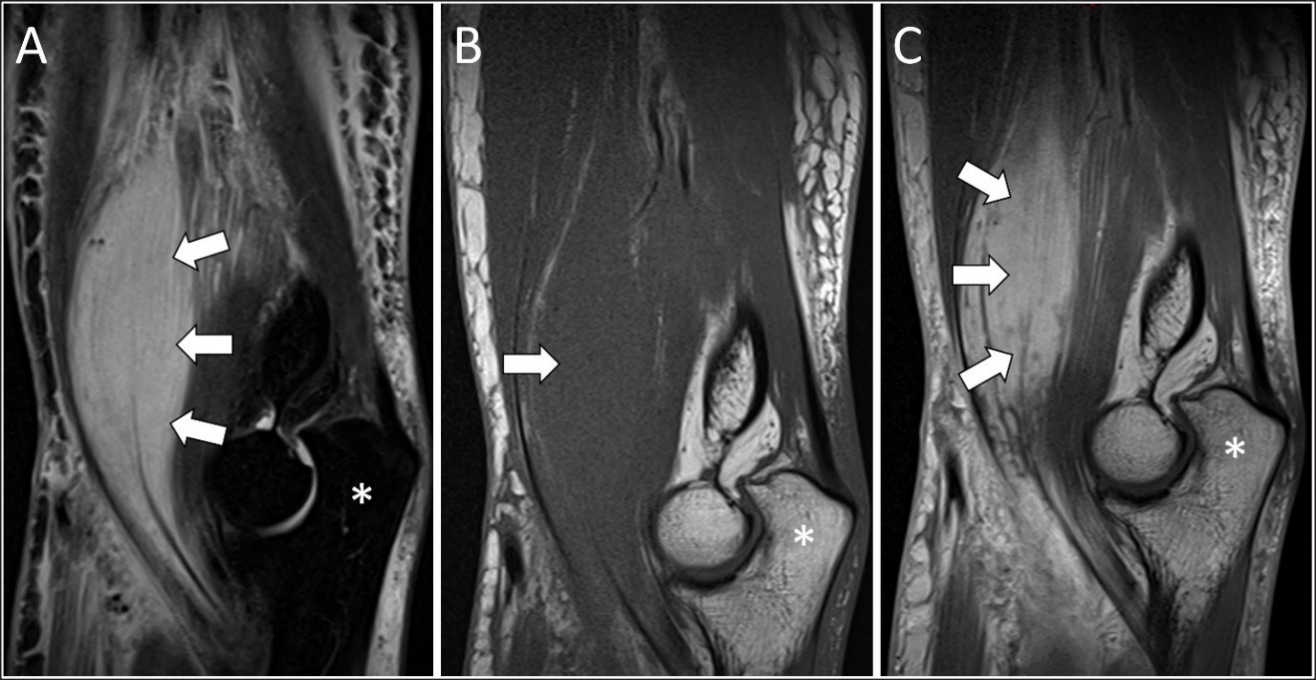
Sagittal MRI images of PM (white arrows) in the right brachialis muscle: hyperintense on STIR images (A), isointense compared to the muscle on T1WI (B), and a marked enhancement on post-contrast T1WI (C). Olecranon (*).
